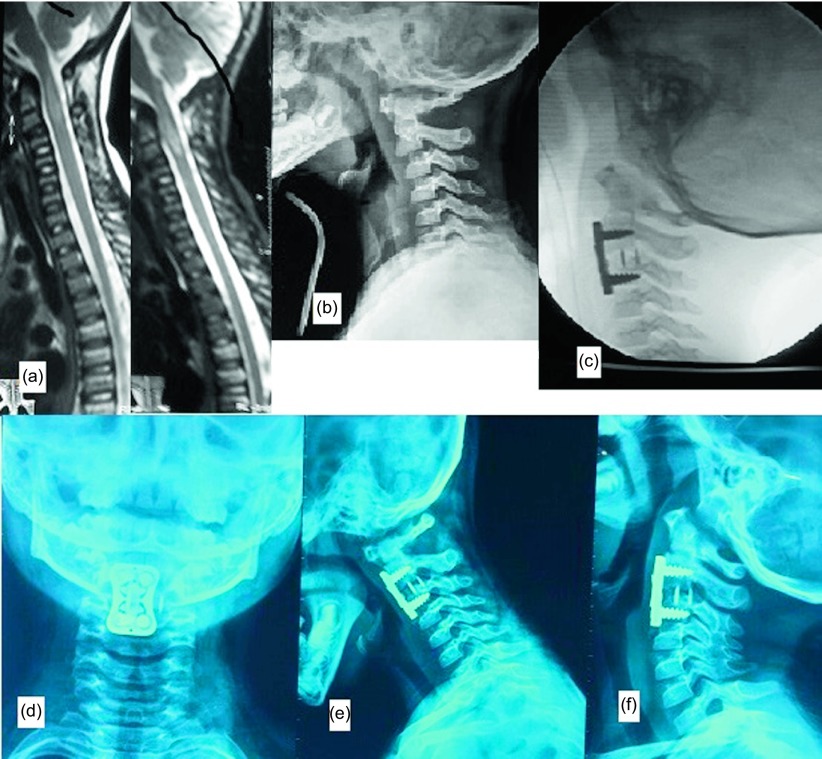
Case 4: A four-year-old girl. (a) Preoperative MRI showing disco-ligamentous injury at C2/3 level with spinal cord edema, (b) preoperative lateral X ray showing C2/3 subluxation, (c) intraoperative radiograph confirming the reduction of C2/3 with fixation using cage and cervical locked plate, (e–g) one-year follow-up anteroposterior (AP), flexion and extension lateral X rays showing solid fusion.
